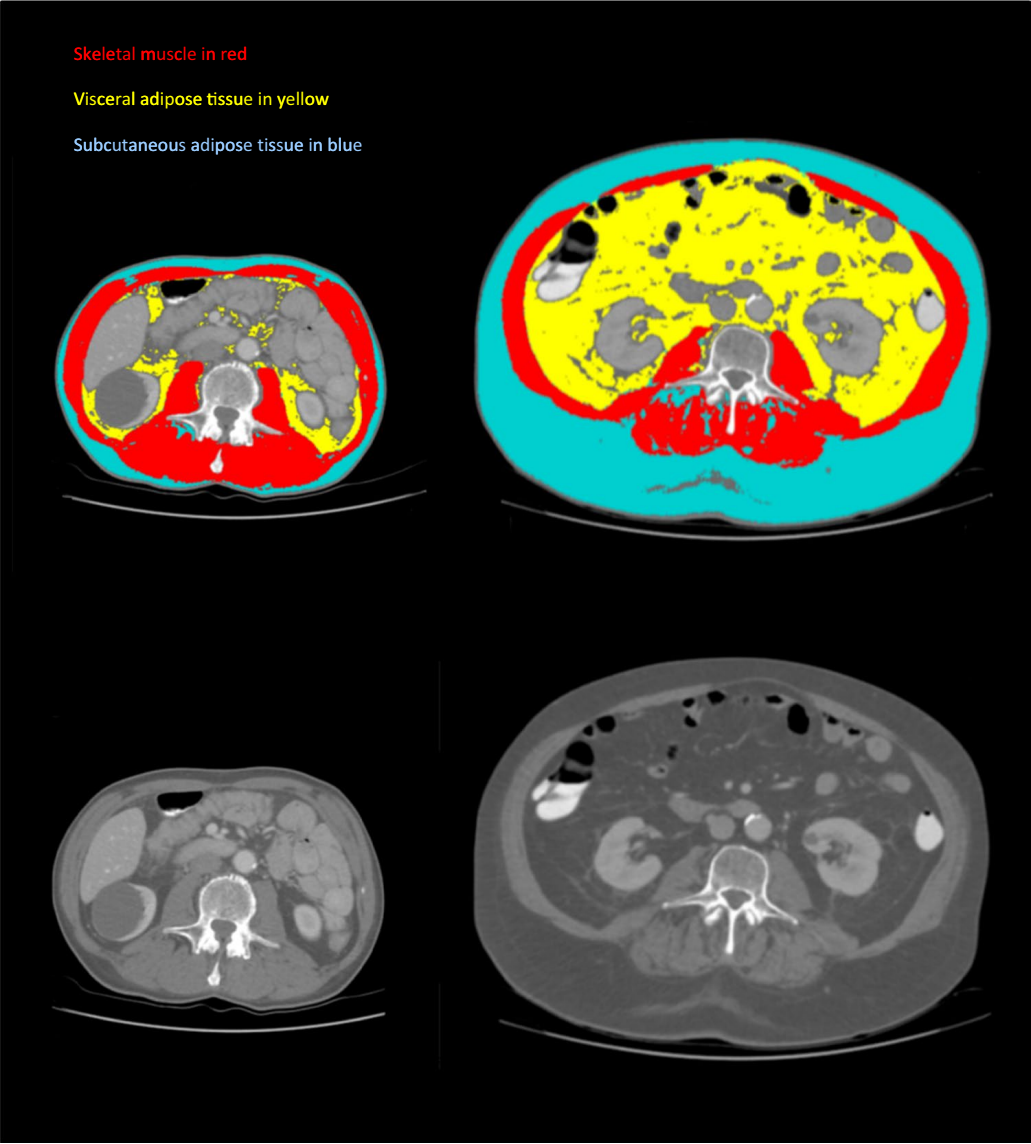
Example CT scans. In the top 2 scans SM, VAT, and SAT are delineated in red, yellow, and blue, respectively. The bottom 2 scans are the same scans without delineations. On the left, a patient is displayed with low *Z*-scores for VAT-M/SAT-M (low amount of fat) and high *Z*-scores for VAT-RA/SAT-RA (lighter shade of gray, indicative of low triglyceride concentration) On the right, a patient is displayed with high Z-scores for VAT-M/SAT-M (high amount of fat) and low *Z*-scores for VAT-RA/SAT-RA (darker shade of gray, indicative of high triglyceride concentration). The body composition of the patient on the left is associated with a higher rate of severe postoperative complications
